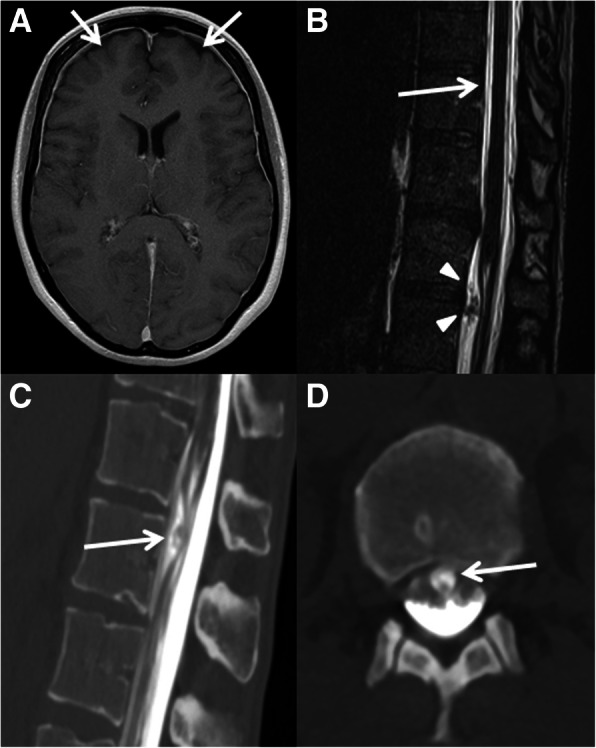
Diagnostic findings in a patient with spontaneous intracranial hypotension (SIH). Initial head-MRI revealed minor bifrontal hygroma (axial view, arrows) with diffuse dural contrast-enhancement as typical findings in a patient with SIH (Panel a). Subsequent whole-spine MRI (sagittal view) shows an extradural fluid collection (arrow) and an unidentified trans−/intradural mass lesion (arrowheads) at the level of thoracic vertebrae 12/lumbar vertebrae 1 (Th12/L1, Panel b). Finally, CT-myelography confirmed the suspected dural leakage caused by the unidentified trans−/intradural mass lesion (arrow) at the level of Th12/L1 (Panel c and d with sagittal and axial slices, respectively)
